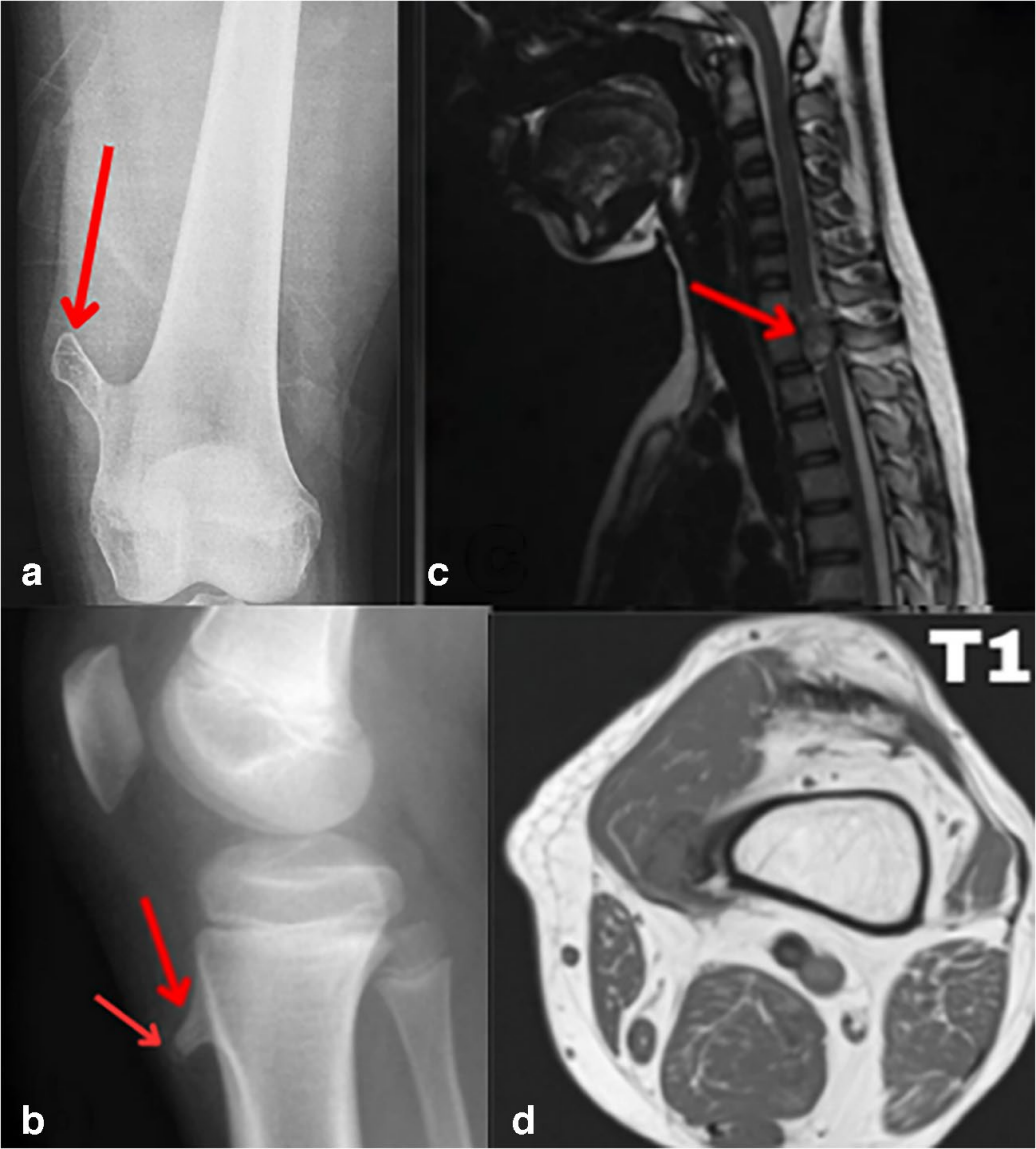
The AP radiograph image of the knee (a) shows a well-defined lesion in the medial aspect of the femoral metaphyses directed away from the joint. The knee joint is normal in appearance. The lesion was hard in nature and found as a lump in the left thigh with the thought of potential continuity, which was disapproved, due to the absence of clear continuity on advanced imaging. The lateral radiograph image (b) reveals a sessile ossific tibial metaphyseal lesion and a small bony protrusion from the anterior metaphysis, separated from the growth plate, with chondroid calcification and no continuity with the adjacent bone marrow, which was initially thought as well to be continuous but appeared on advanced imaging otherwise. A second arrow points to the chondroid calcification found at the anterior margin of the lesion. A STIR MR of the cervical spine in image (c) demonstrates a well-defined lesion extending into the spinal canal, causing mass effect and early myelopathic changes in the dorsal spinal cord; STIR MRI was performed specifically in this case due to its capability to suppress fat signals, which was essential for visualizing the osteochondromatous lesion without interference from overlapping fat signals. Posterior scalloping of the T2 vertebral body is also evident, highlighting the lesion’s pressure impact and remodeling effects. The T1-weighted MRI shown here in (d) demonstrates the lack of continuity of the lesion in the distal femur with the medulla. The lesion’s low intensity on the T1 image is also evident
